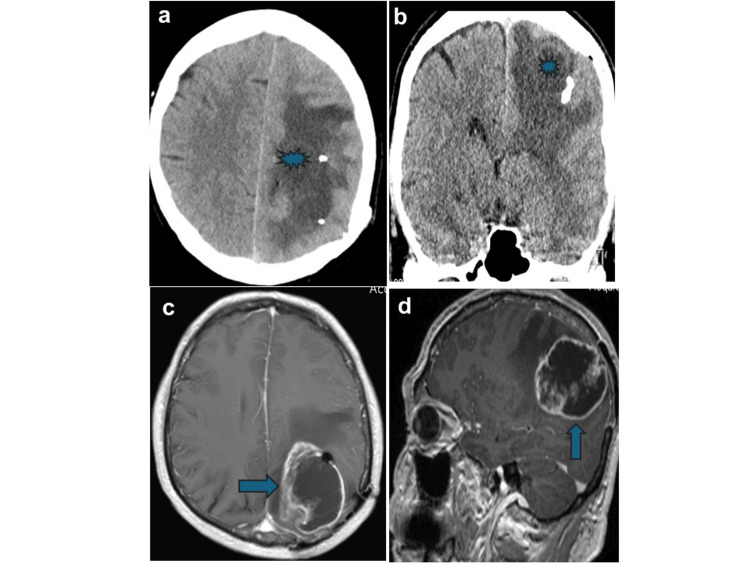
Preoperative cerebral imaging. (a, b) Native axial and coronal CT images showing cerebral edema and a suspected lesion in the left parietal region. (c, d) Axial and sagittal T1-weighted MRI sequences with gadolinium contrast showing a contrast-enhancing lesion with surrounding edema in the left parietal region.
